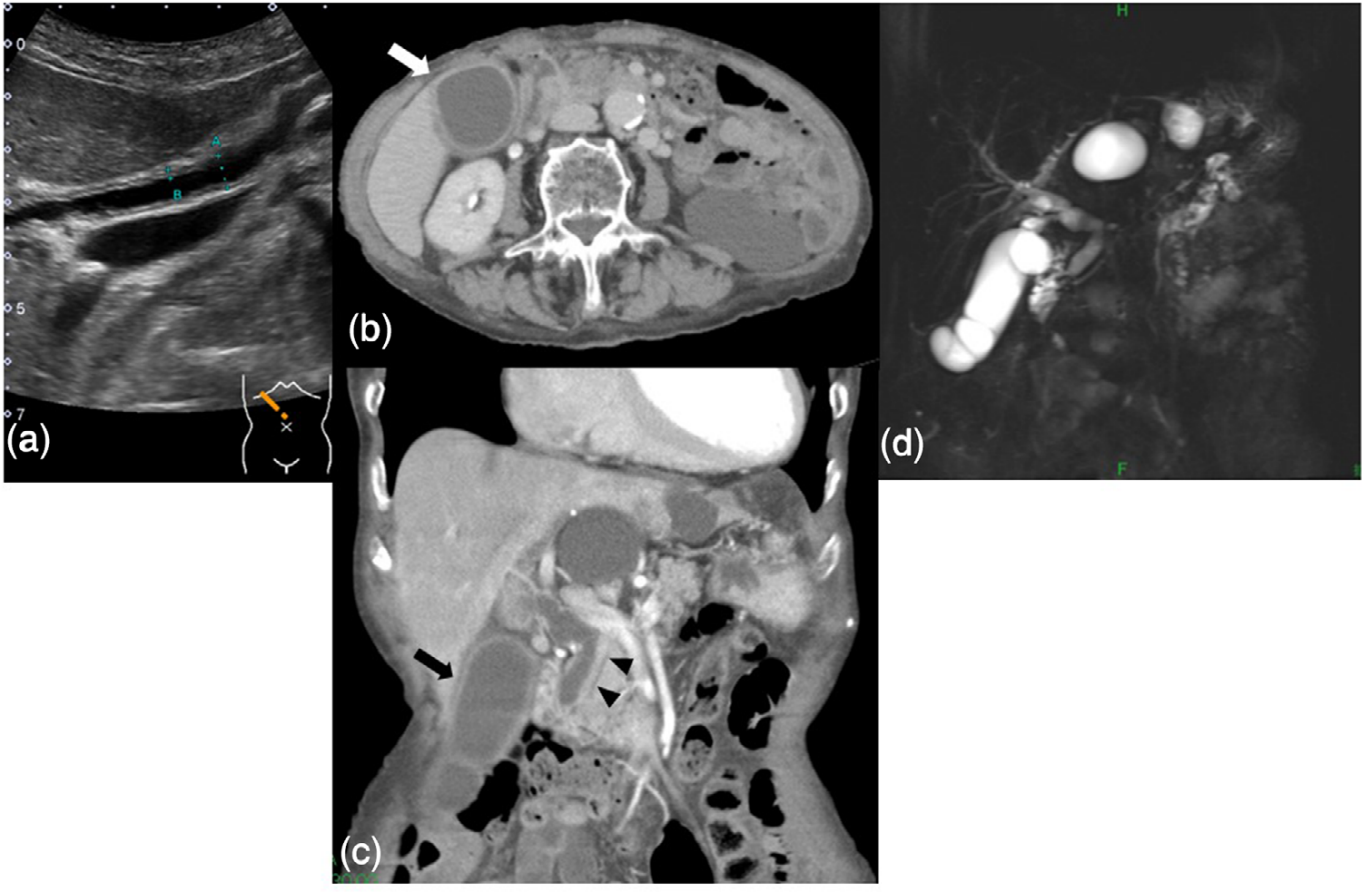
(a) Abdominal ultrasonography showing the diameter of the common bile duct was 6.3 mm (A) and the thickness of the common bile duct wall was 1.3 mm (B). (b, c) Contrast‐enhanced computed tomography of the abdomen showing enlargement and edematous thickening of the gallbladder (→) and bile duct walls (▲). The bile duct dilatation was mild, and no stones were observed. (d) Magnetic resonance cholangiopancreatography showing that the common bile duct was dilated. However, there was no dilatation and stenosis of the intrahepatic bile ducts
